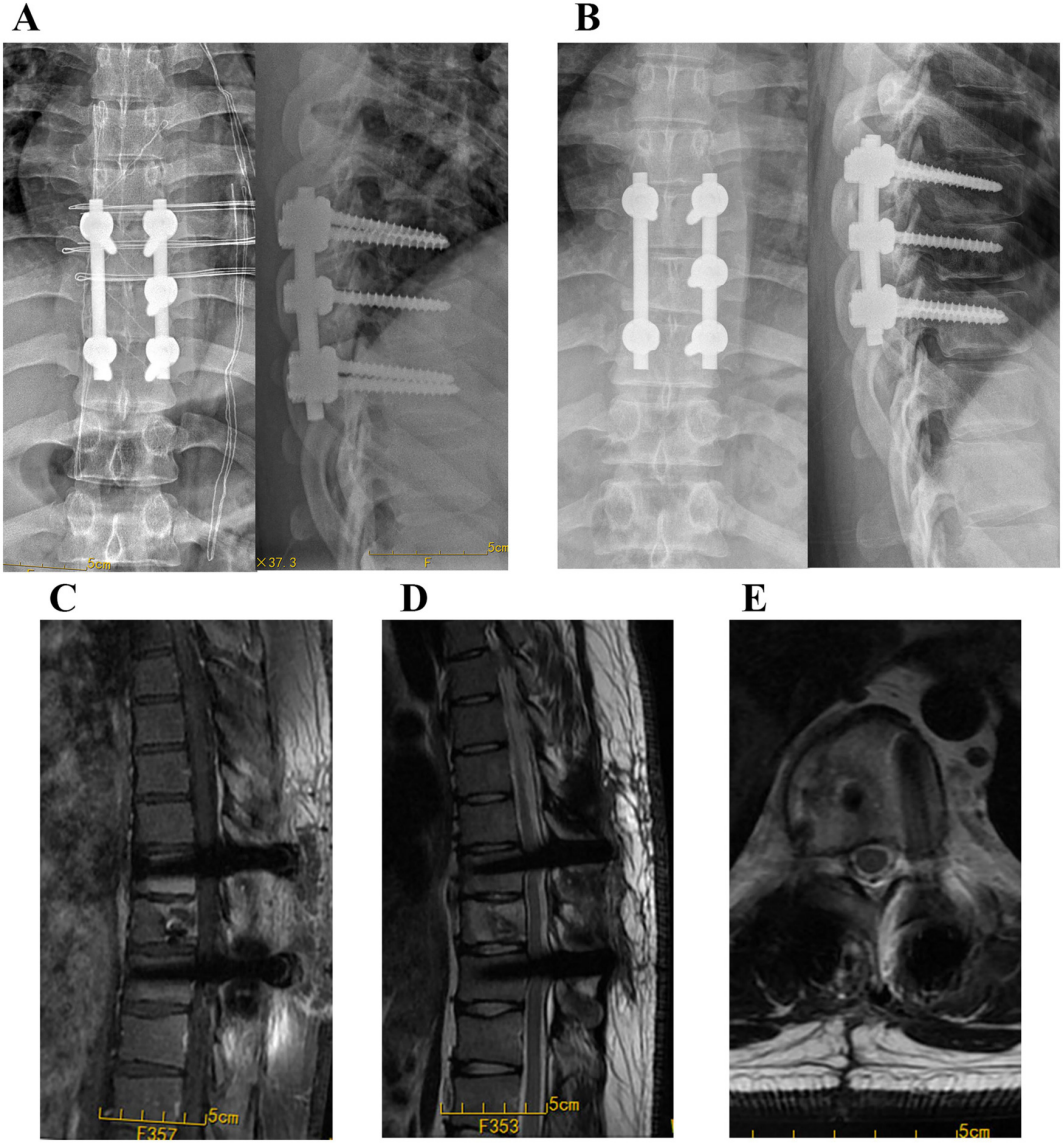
Postoperative follow-up imaging examination. (A) The X-ray of the spine at the 1 month postoperation. (B–E) The X-ray and MRI images at the 6 months postoperation.
